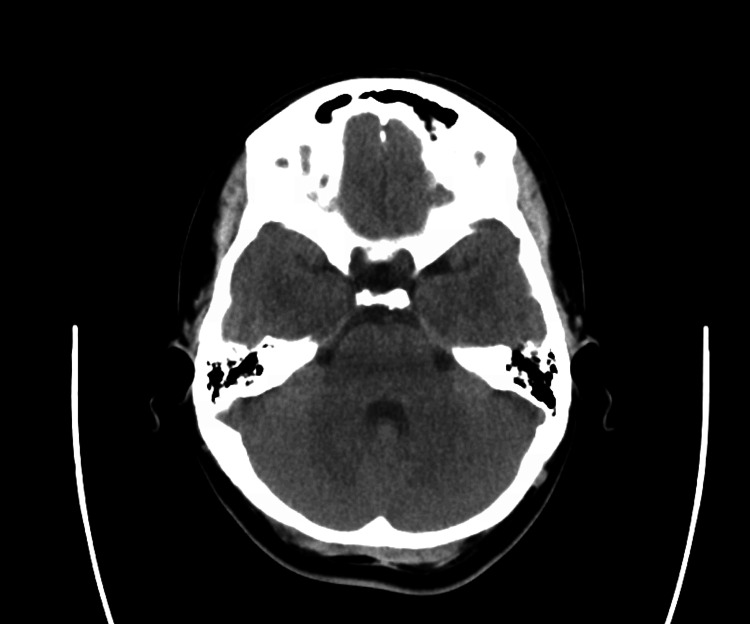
CT head (transverse view)
